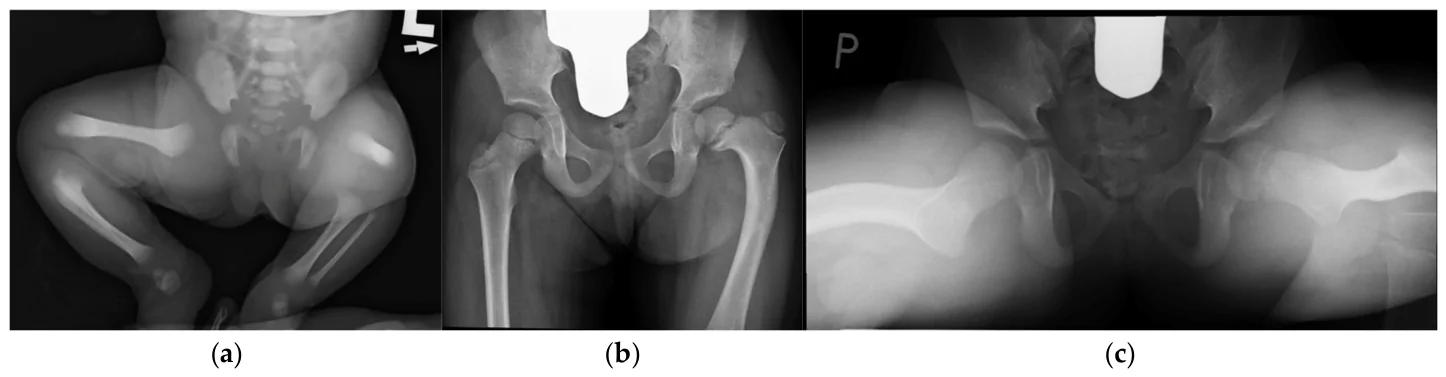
Radiographic views of the lower limb in a patient with right femoral shortening: (a) radiograph obtained just after birth; (b) pelvic radiograph at 3.5 years of age showing CFD type 1A3; (c) Rippstein position radiograph demonstrating proximal femoral retroversion. P—right.
